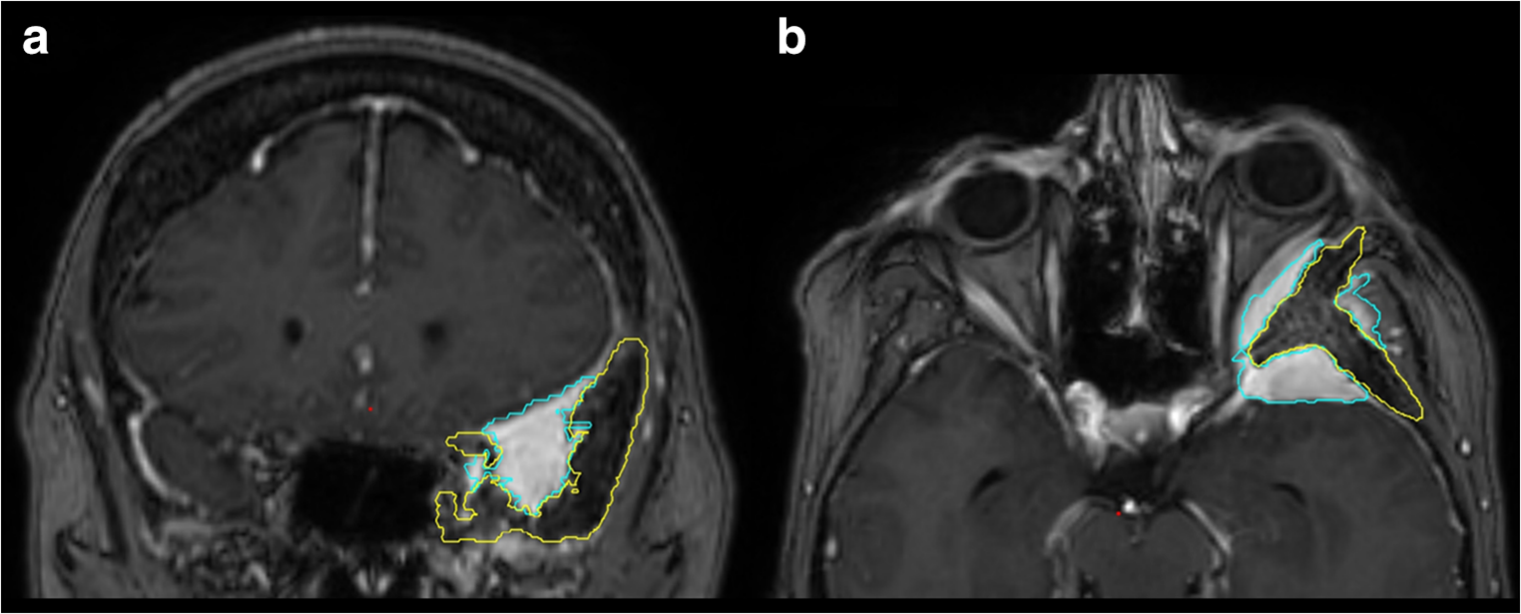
Case 49f, left-sided SOM: intraoperative multimodality image guidance concurrently displaying (1) osseous tumor extension (CT-based segmentation, *yellow*) and (2) soft tumor extension (MR T1 CE-based, *blue*). The background image is a MR T1 CE fat suppression MRI. a Soft tumor extension into the orbit via the SOF with surrounding osseous SOM involvement of the anterior clinoid process, the middle fossa floor extending up to the lateral wall of the sphenoid sinus, and the temporal bone. b Soft tumor extensions at the temporopolar dura, the SOF, the lateral orbit, and the temporal muscle. Osseous tumor extension in the greater wing of the sphenoid bone
